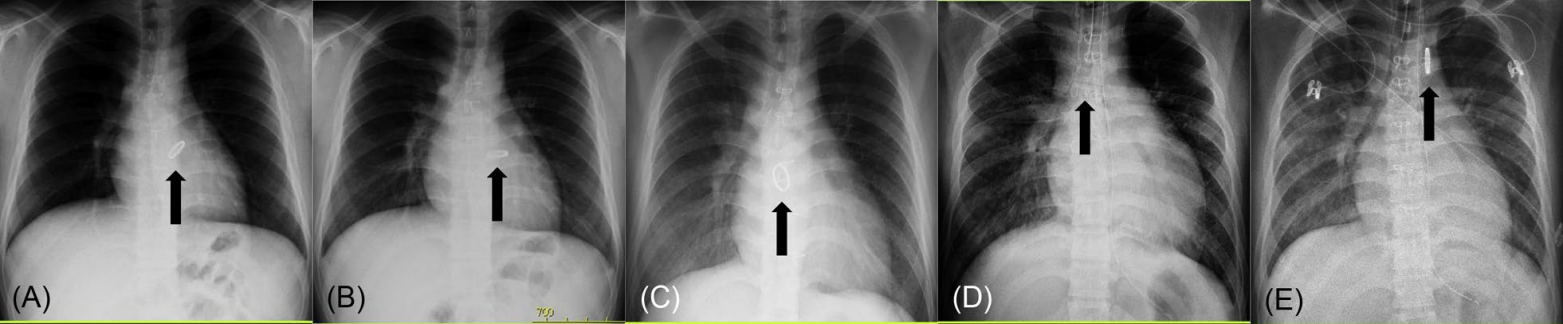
Chest X-ray findings 3 months ago (A), 1 week ago (B), at emergent admission to the regional hospital (C), at leaving for our hospital (D), and at arriving at our hospital (E). Black arrows indicating mechanical valve
